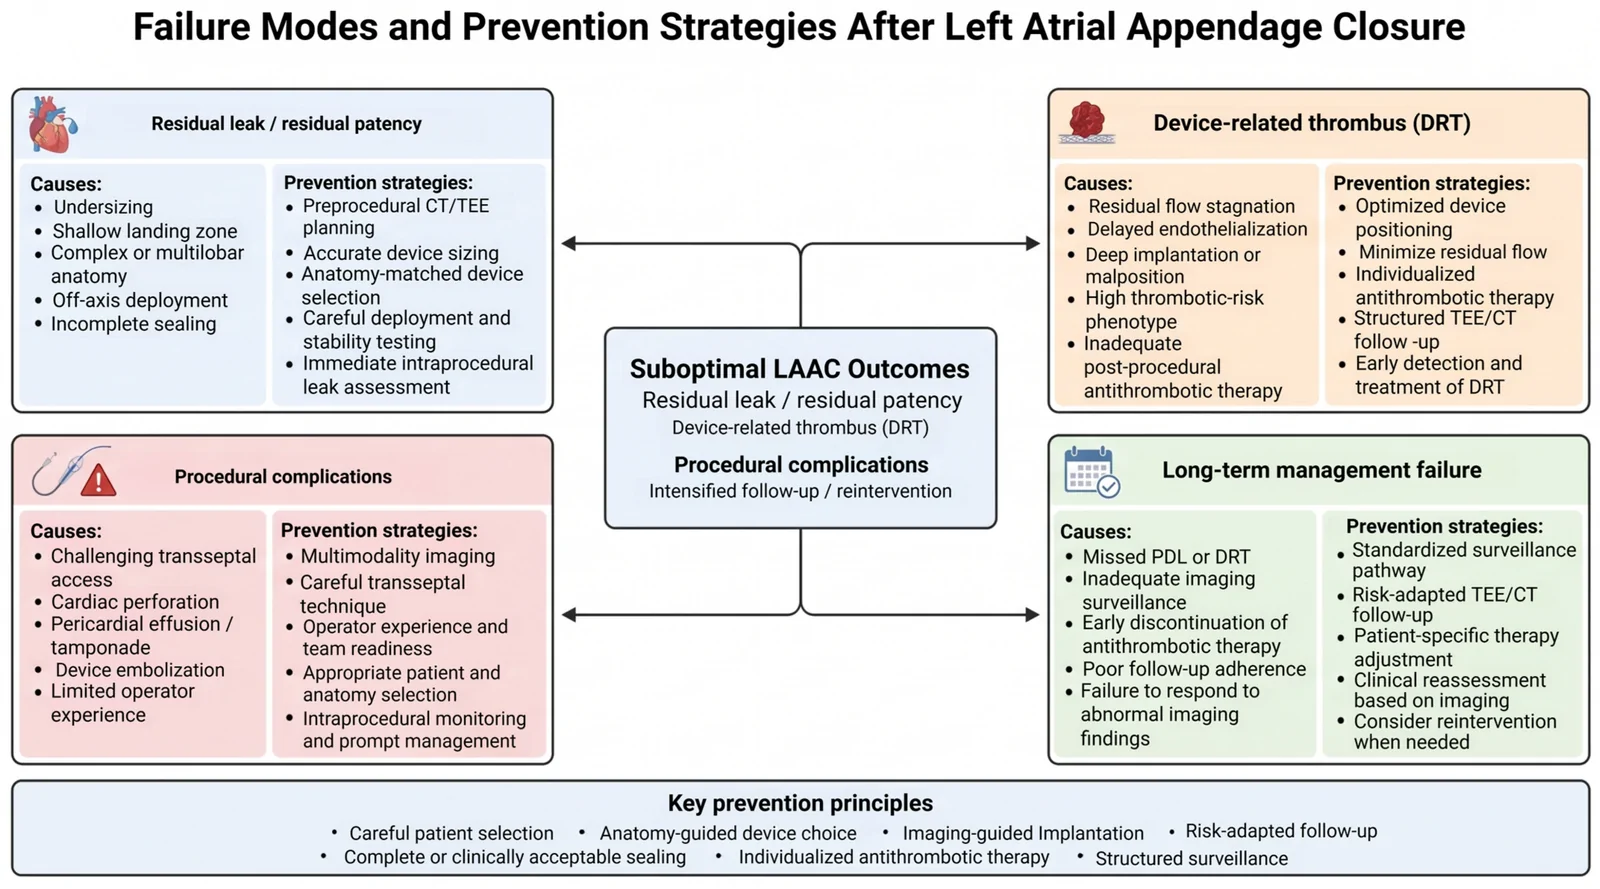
Suboptimal LAAC outcomes may result from residual leak or residual LAA patency, device-related thrombus, procedural complications, and inadequate long-term management. These risks may be reduced through careful patient selection, anatomy-guided device choice, multimodality imaging, optimized implantation and sealing, individualized antithrombotic therapy, and structured surveillance. Abbreviations: CT, computed tomography; DRT, device-related thrombus; LAAC, left atrial appendage closure; PDL, peri-device leak; TEE, transesophageal echocardiography.
